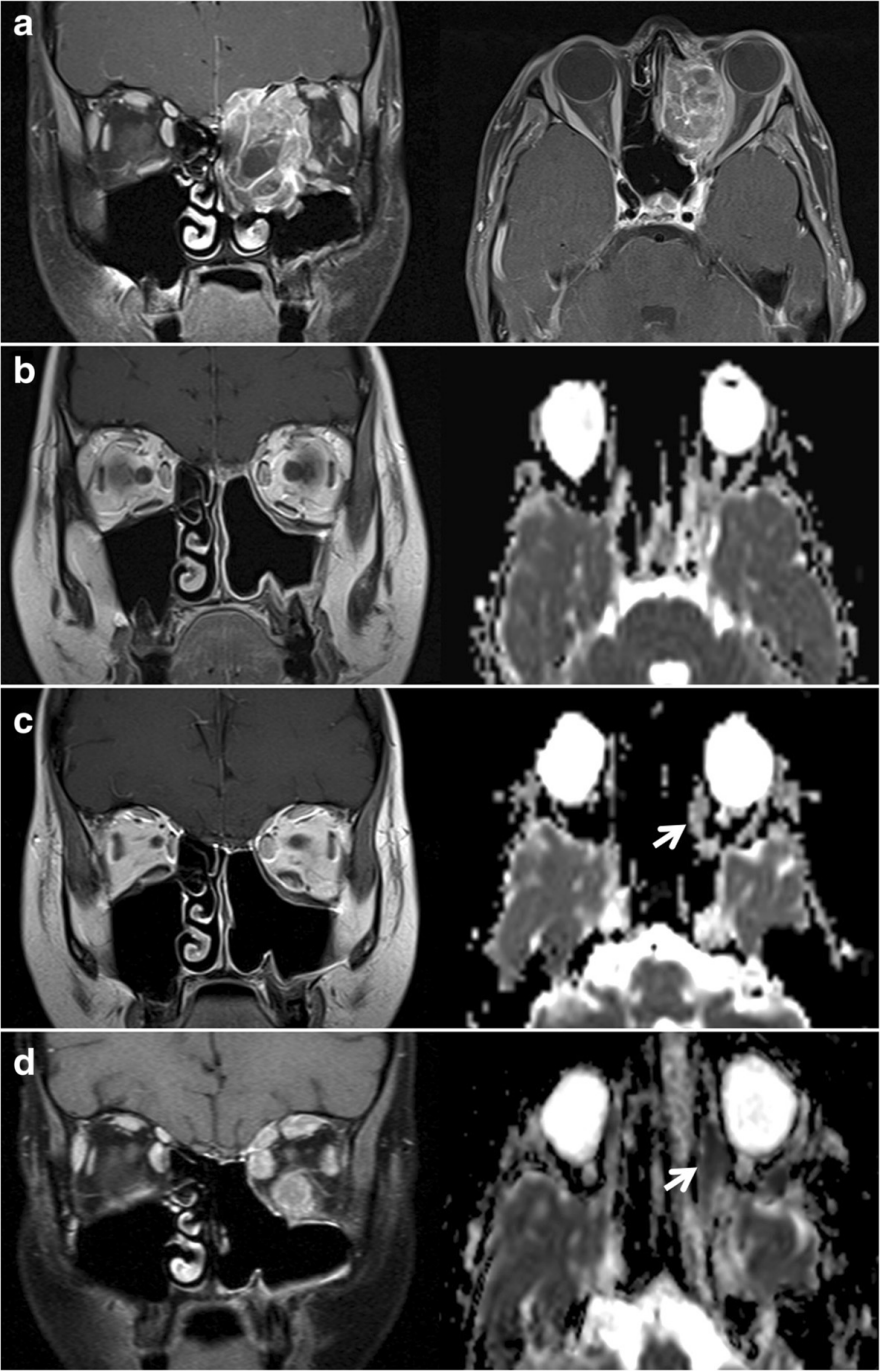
Longitudinal MRI studies of the orbitae. (a) Initial diagnosis of the left-orbital rhabdomyosarcoma with infiltration of the ethmoidal air cells and the left maxillary sinus (coronal and transversal contrast-enhanced fat-saturated T1w). (b) Normal post-therapeutic follow-up study. (c) At onset of double vision, discrete thickening of the left medial and inferior rectus muscle in the coronal T1w and of the left lateral rectus muscle in the ADC-map (arrow) became apparent, yet without pathological decrease in diffusivity (ADC = 1.25 x10^−3^ mm^2^/s). (d) Follow-up MRI showed progressive signal alterations in all left extraocular eye muscles and initial changes in the right medial rectus muscle. Both left and right medial rectus muscle showed significantly restricted diffusion (arrow, ADC = 0.62 x10^−3^ mm^2^/s). Figures 1b,c,d show coronal contrast-enhanced T1w and ADC-maps calculated from transversal DWI with b-values of 0 and 1000 s/mm^2^. The examinations were performed at 1.5 Tesla (1a and 1d) and at 3 Tesla (1b and 1c).
